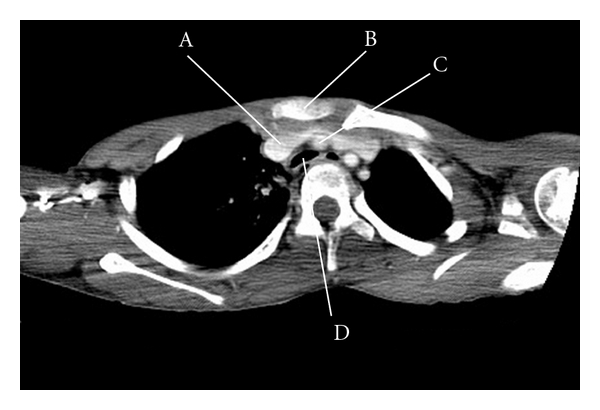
Chest computed tomography. Computed tomogram before the operation shows extrinsic compression of the trachea by the innominate artery. (A) Superior vena cava; (B) manubrium; (C) innominate artery; (D) trachea.
